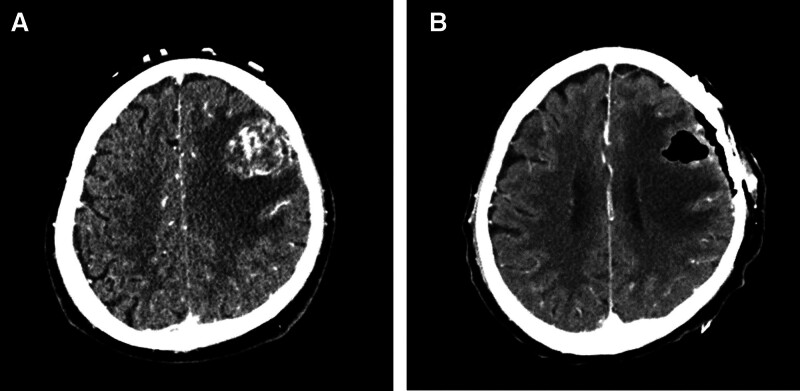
Enhanced brain CT. (A) An enhanced brain computed tomography (CT) scan showed a rim-enhancing mass with vasogenic edema in the left frontal lobe. (B) Follow-up CT after surgical mass removal. CT = computed tomography.
